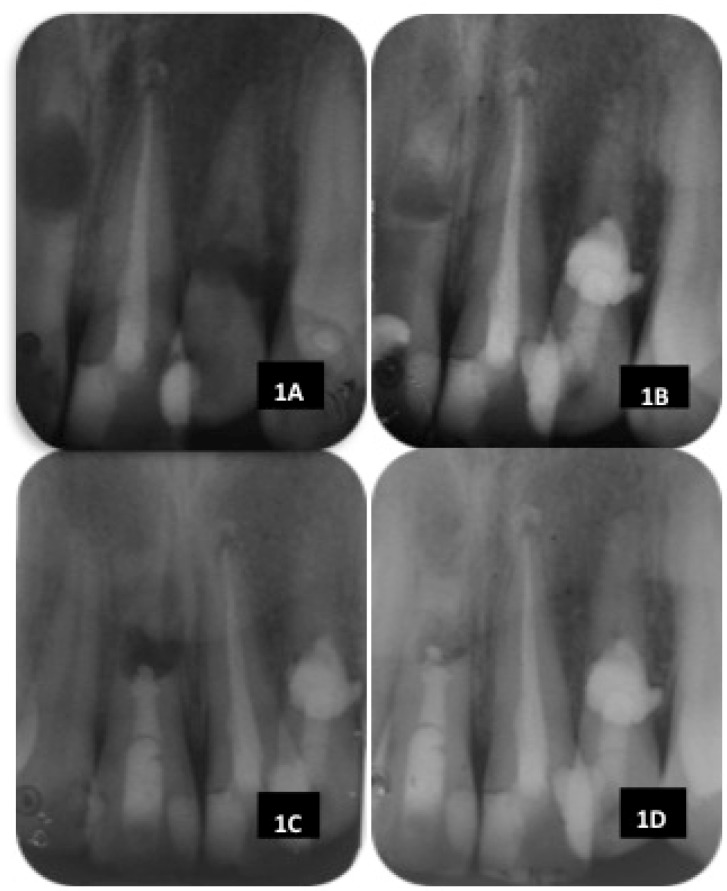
A) Preoperative radiograph; B) One-year review of tooth 22; C) Postoperative of tooth 11 filled with MTA; D) Nine months review post-surgery of tooth 11
